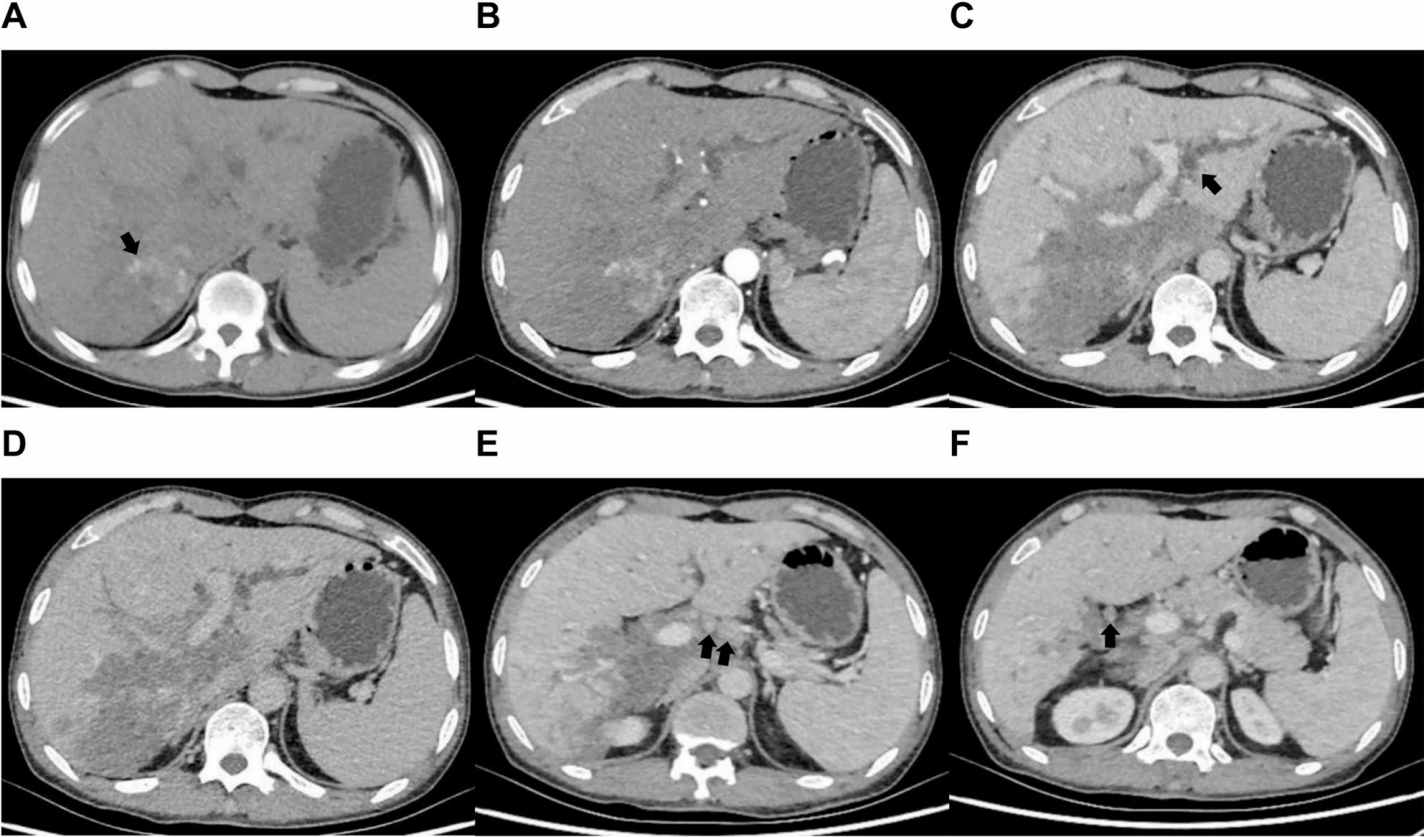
CT findings. (A) The plain CT scan revealed a substantial mixed low-density area in the right lobe of the liver, with hyperdense foci (arrow) within the lesion and encasement of both the right and left portal. (B, C,D) The arterial phase (B), portal veous phase (C), and delayed phase (D) of the lesion on contrast-enhanced CT demonstrated no evident enhancement within the lesion; however, there was notable dilation of the intrahepatic bile ducts (arrow). (E, F) The contrast-enhanced CT images at the level of the porta hepatis show multiple enlarged perihilar lymph nodes (arrows)
